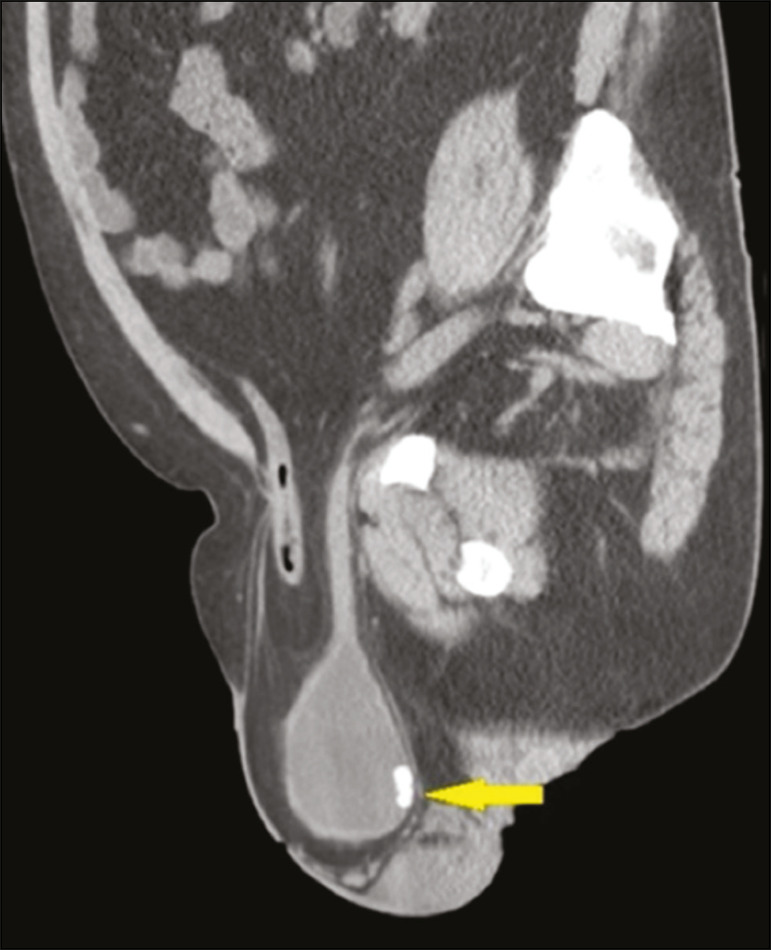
Unenhanced sagittal CT showing a hernia containing an intestinal loop and part of the bladder, together with a calculus (arrow) in the herniated portion.
